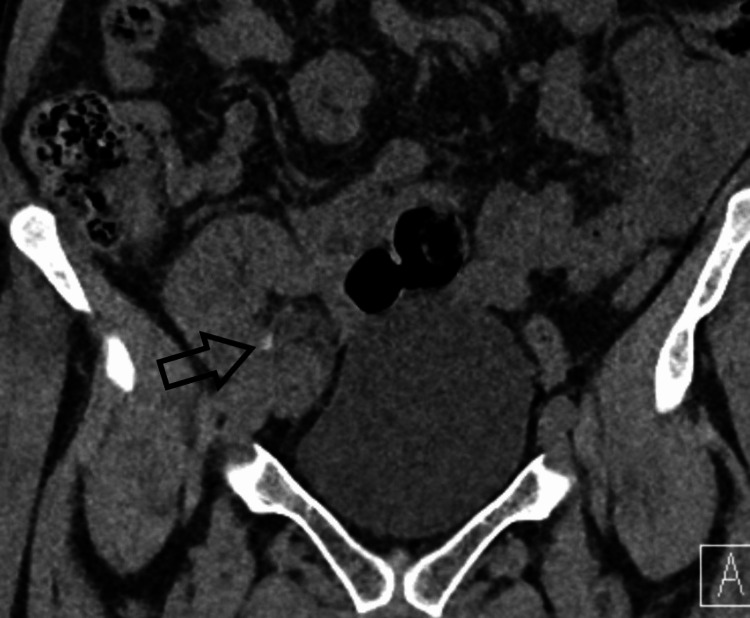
CT non-contrast phase of transplanted kidney showing high-density material in renal pelvis
